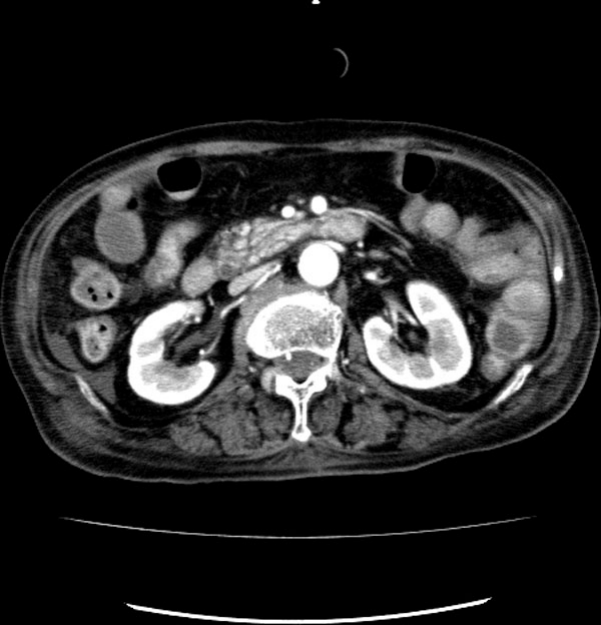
Axial CT scan obtained with IV contrast material in 80-year-old female subject diagnosed with ischemia.
